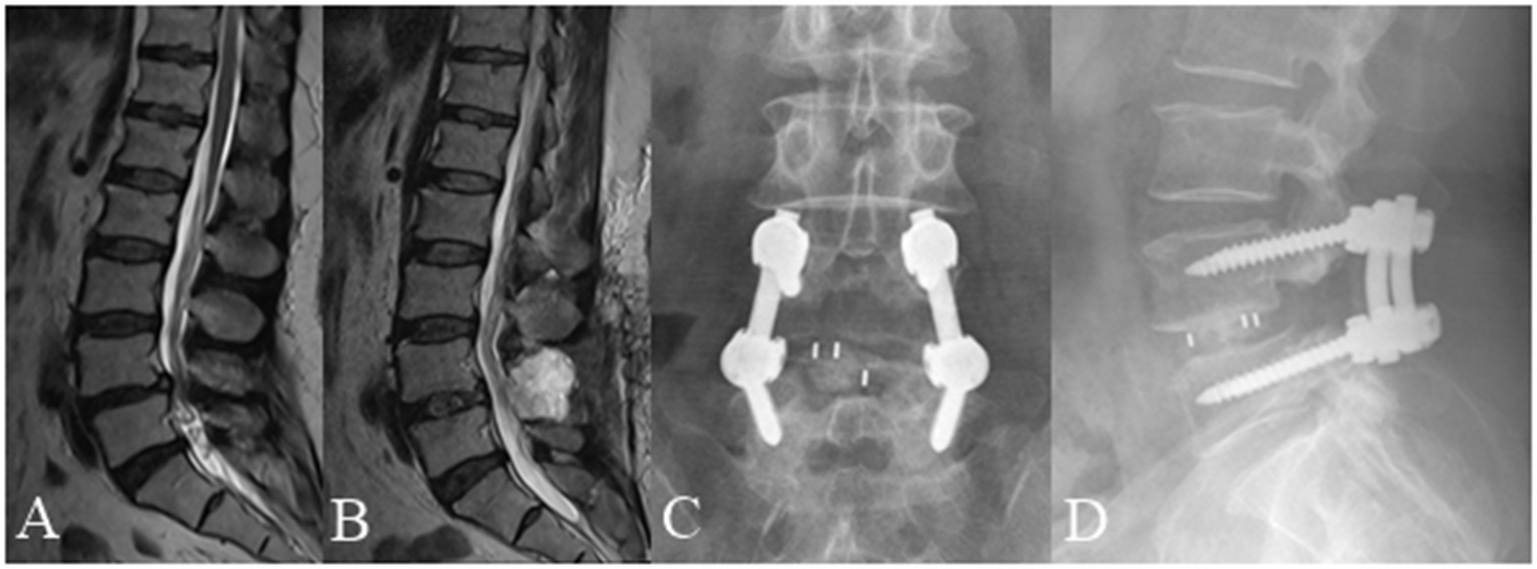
A 59 year old female complained of low back pain and left and right lower extremity pain for 3 months underwent posterior lumbar interbody fusion at L4/5. (A) Pre-operative lumbar MRI showed that the nerve roots were redundant below the L4/5 intervertebral disc. (B) Post-operative MRI obtained 3 days after surgery showed significant improvement with resolution of RNRs. (C,D) Lumbar radiographs obtained 3 months after surgery demonstrate internal fixation in good position without cage subsidence or migration.
